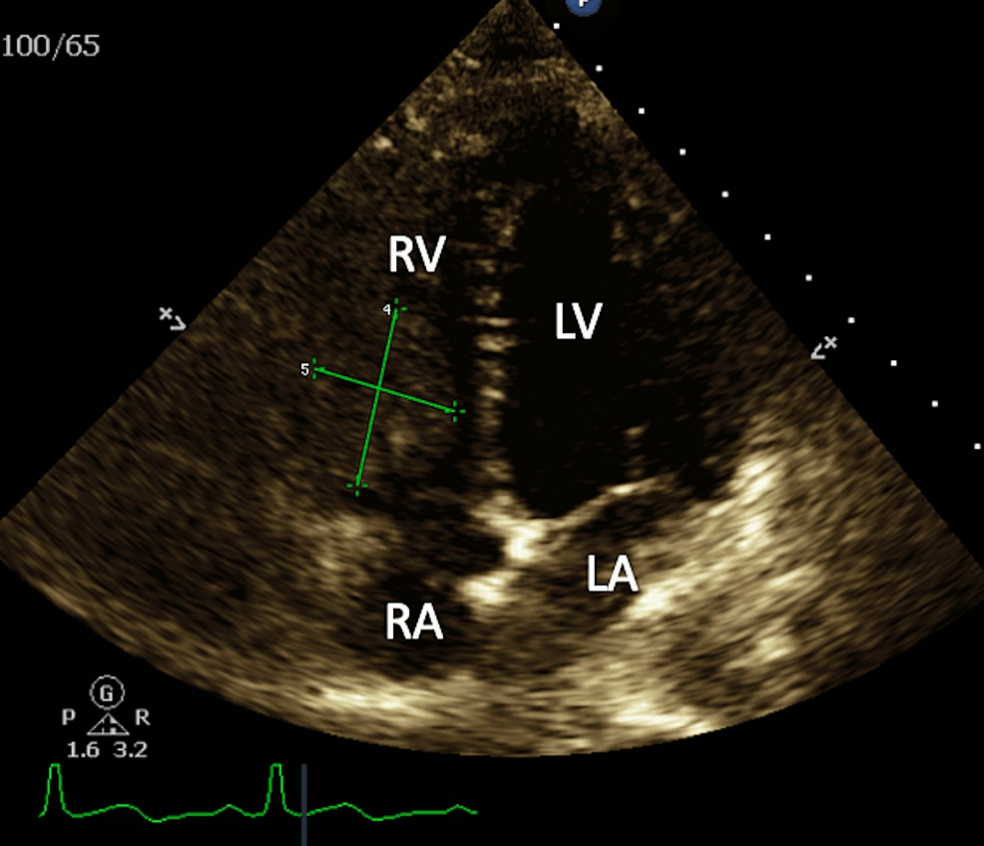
A four-chamber view of transthoracic echocardiography showing a right ventricular mass. RV: right ventricle; LV: left ventricle; RA: right atrium; LA: left atrium
